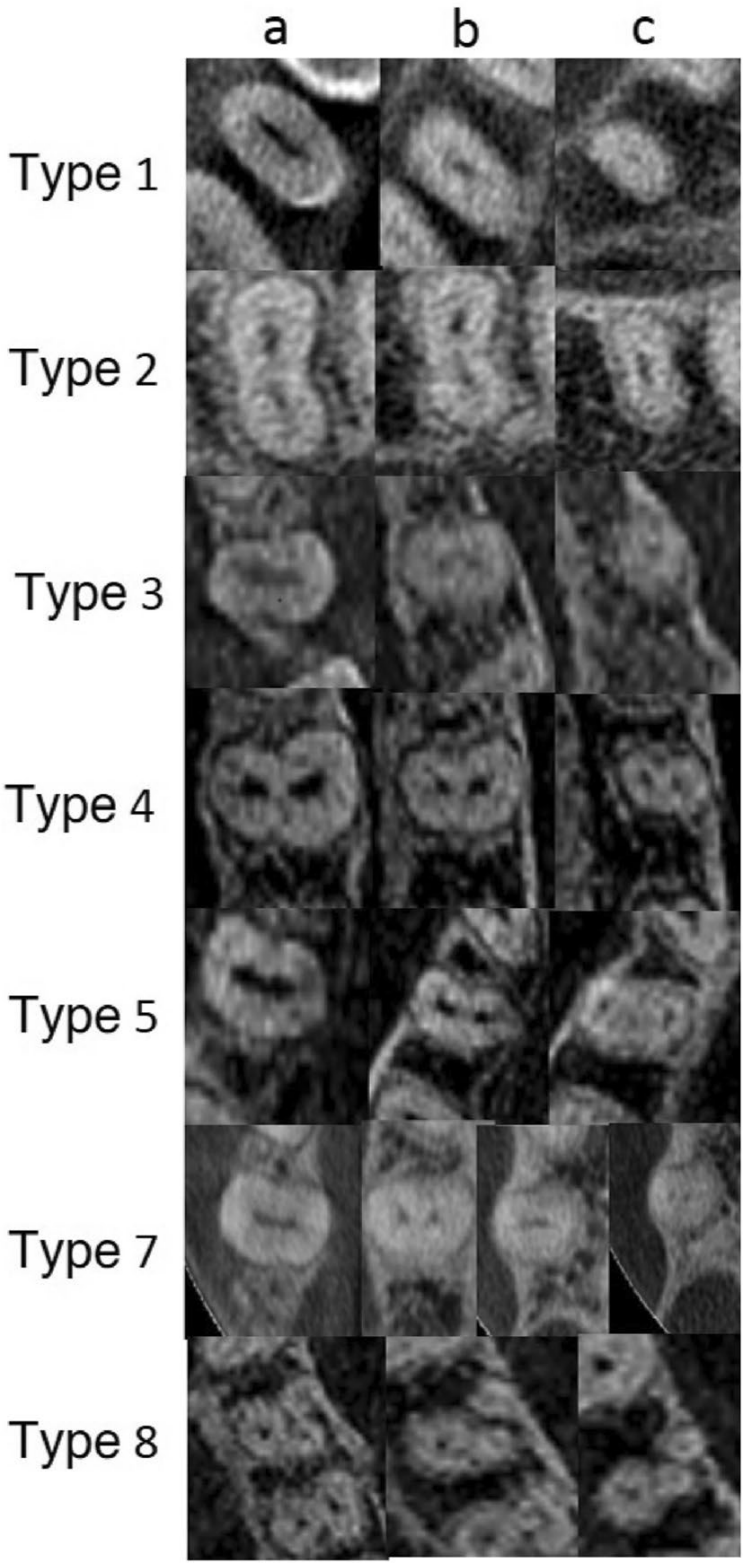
CBCT scanning in the horizontal plane of the coronal (a), middle (b), and apical thirds (c) of the root demonstrated variations in canal morphology according to Vertucci classification
